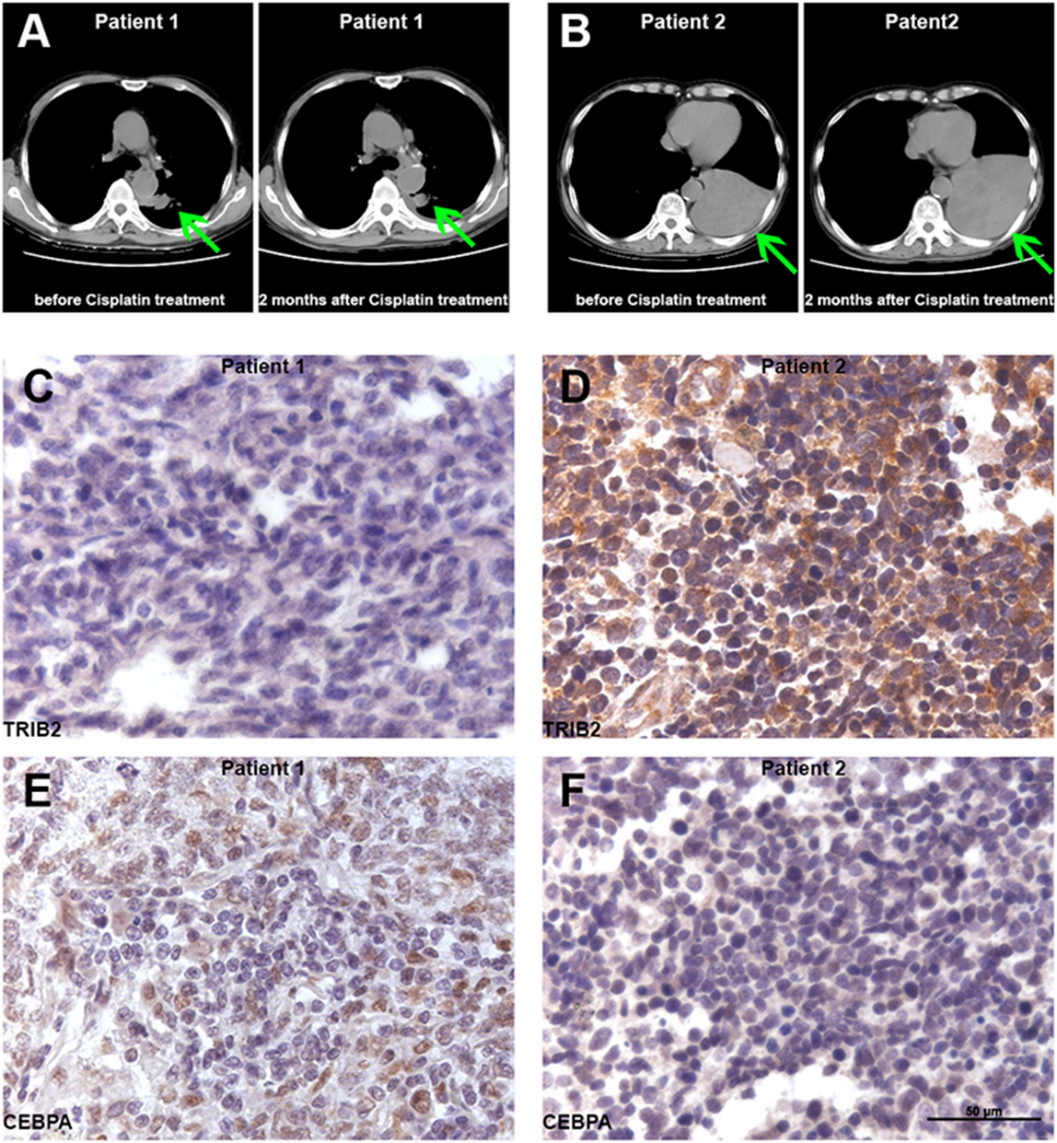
TRIB2 and CEBPA proteins in SCLC patients (A) Patient 1: CT scan before and two months after Cisplatin treatment. (B) Patient 2: CT scan before and two months after Cisplatin treatment. (Arrows indicate tumor.) (C) Tumor sample from the Cisplatin-sensitive Patient 1 did not express TRIB2 protein. (D) Tumor sample from the Cisplatin-resistant Patient 2 presented high levels of TRIB protein in the cytoplasm and nucleus of tumor cells. (E) Tumor sample from Patient 1 demonstrated CEBPA protein in the nucleus of the tumor cells. (F) Tumor sample from the Patient 2 did not have detectable CEBPA protein levels.
